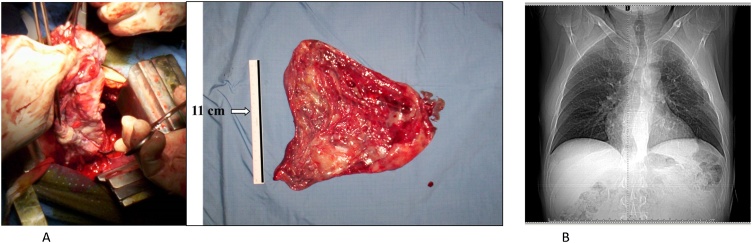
A. Surgical isolation of the giant bulla. The bulla measured 11 cm in length. 3B Six-day postoperative chest X-ray.
